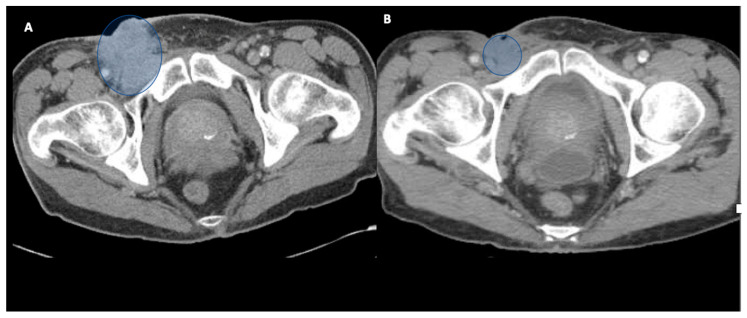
Computerized tomography (CT) scan images from baseline (A) and post treatment (B). Time points represent significant decrease in size of necrotic right inguinal nodal mass infiltrating the muscle, originally 5.3 × 5.0 cm prior to radiation therapy (RT).
